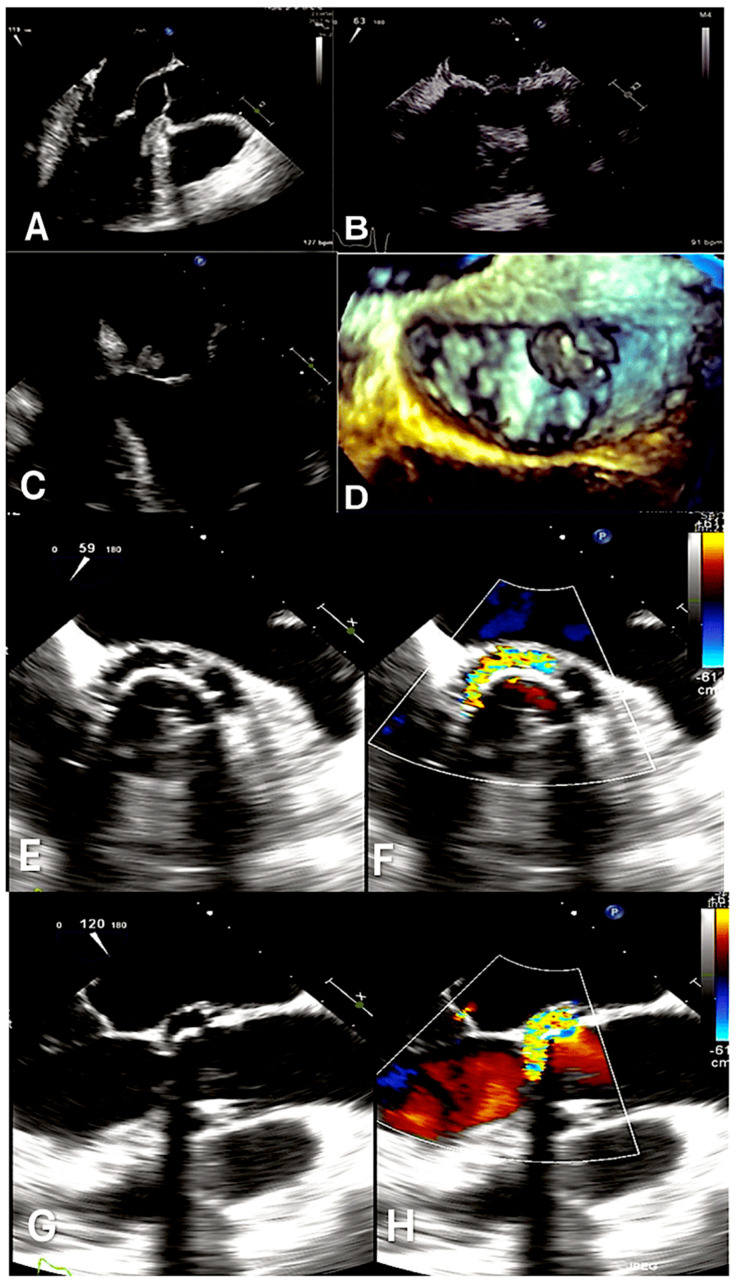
The following section reviews the echocardiographic findings in mitral valve endocarditis. (A) depicts a representative example of the normal mitral valve in two-dimensional transesophageal echocardiography, with the probe rotated to 120°. The thinner leaflets of the mitral valve are visible in the open position, in mid-diastole, with the aortic valve in the closed position. The left atrium is of an appropriate size, without any enlargement, and the left ventricular wall thickness is also within normal limits. (B) Mitral xenograft endocarditis. (C) Two-dimensional transesophageal echocardiography displays a vegetation on the atrial aspect of the anterior leaflet. (D) Three-dimensional reconstruction of the mitral valve (surgeon’s view) depicts the vegetation in C. Prosthetic valve endocarditis is evident. (E,F) The images presented are those obtained from the short-axis view (59 degrees of probe angle) and (G,H) the long-axis view (120 degrees). The images are presented in two different ways: with (A) and without color-Doppler analysis. The color doppler analysis was performed on a mechanical aortic prosthesis with a posterior semilunar abscess, which involved the aortomitral junction.
